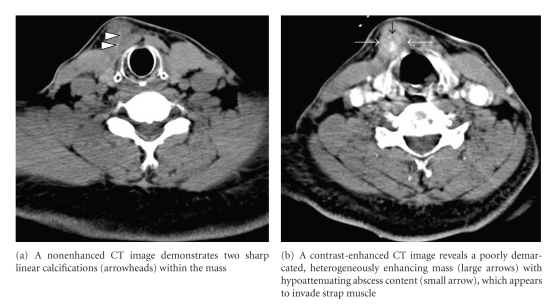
CT features of fishbone-associated actinomycosis of the right anterior cervical space.
